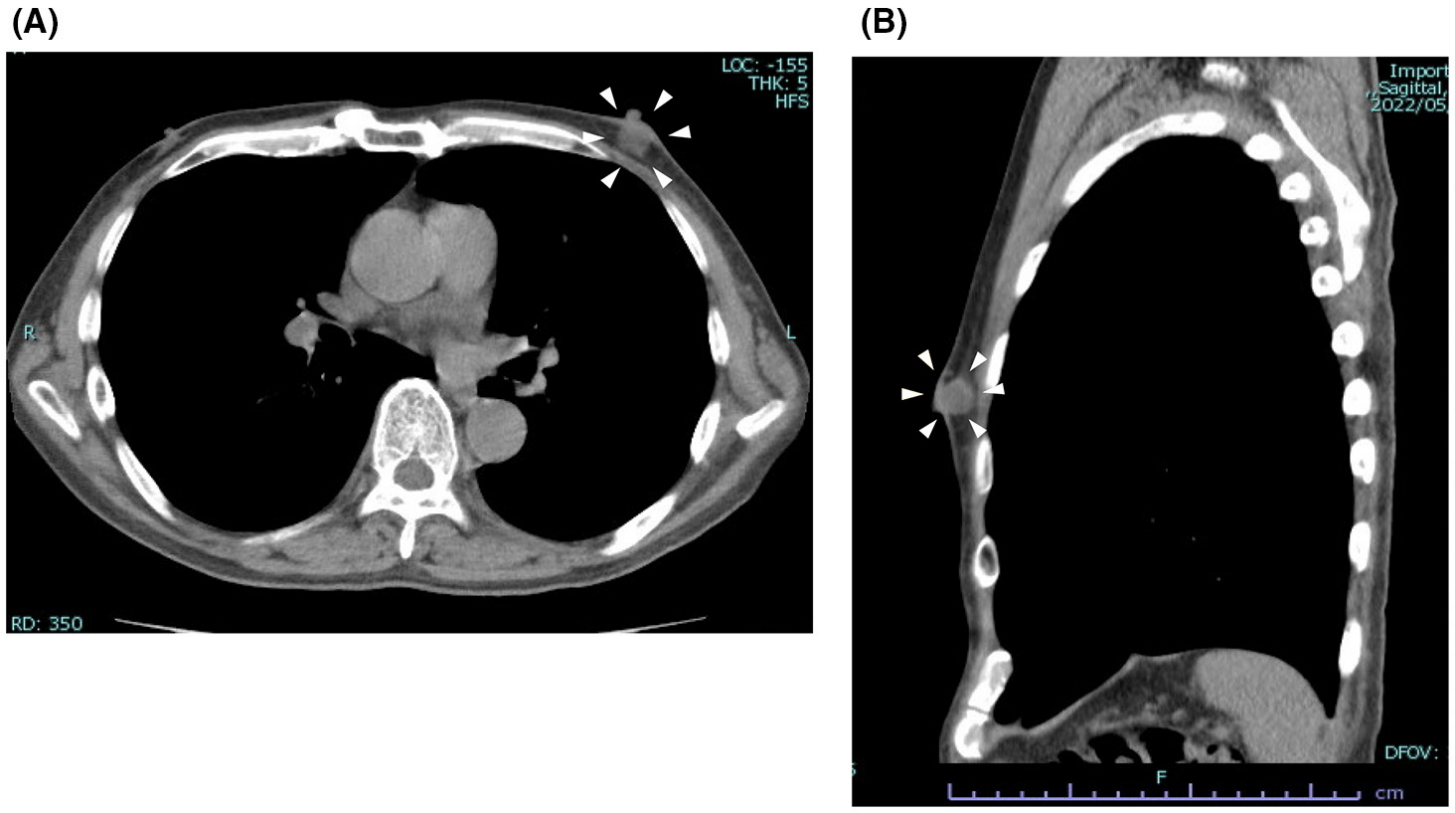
Plain computed tomography scan (A) coronal plane, (B) axial plane at the first visit. A tumor measuring 13.9 × 12.9 × 6.1 mm in the subareolar portion of the left breast (Arrowhead).
